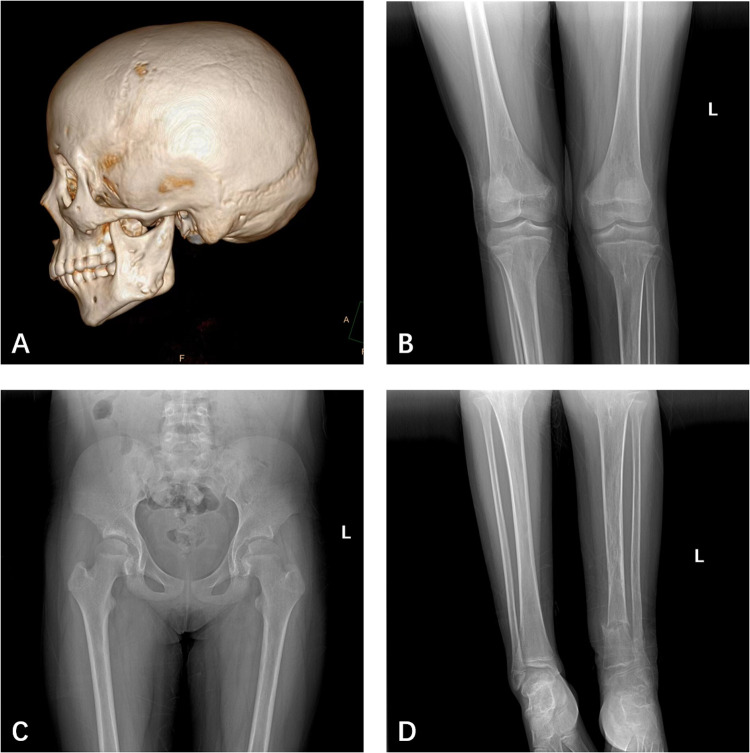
(A) CT showed bone abnormalities in the left parietal bone, and left mandible. (B) X-ray showed bone abnormalities in both femurs, tibia, and fibula. (C) X-ray showed bone abnormalities in both femurs, but not the iliac bone. (D) X-ray showed the left distal tibia and fibula fractures and nonunion.
